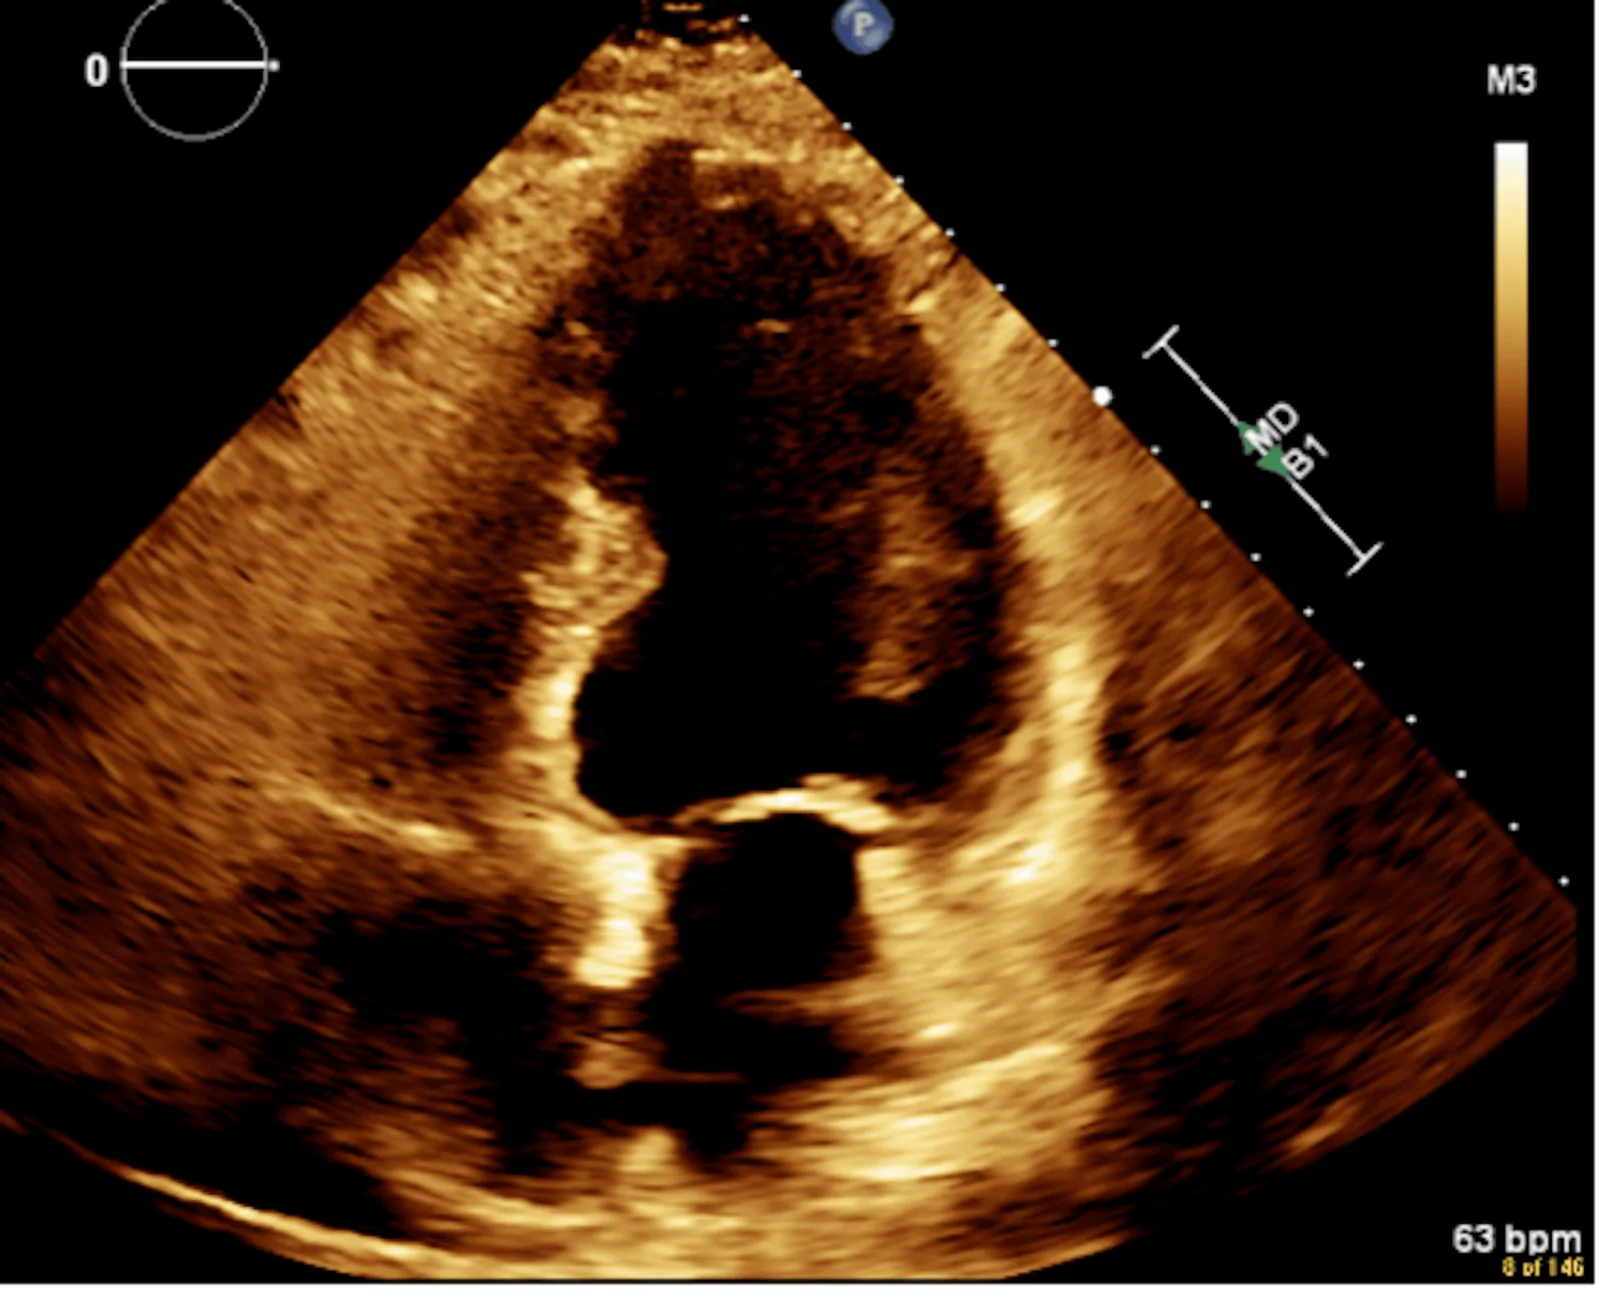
Repeat TTE in April 2026 without ultrasound contrast agent (A4C view) Apical thrombus still present TTE: transthoracic echocardiography, A4C: apical four-chamber, LV: left ventricle
